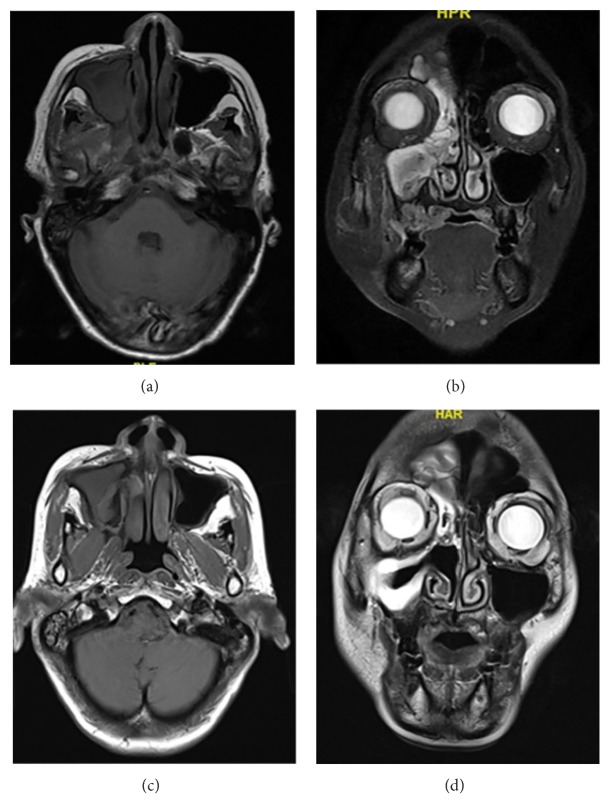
A 64-year-old woman was diagnosed with IMT of the nasopharynx and paranasal sinus. She underwent incomplete resection of tumor, combined with oral prednisone and radiotherapy. After 20-month follow-up, both clinical and imaging manifestations improved. The axial and coronal MRI images before treatment were shown above ((a) and (b)) and that 9 months after radiotherapy were shown below ((c) and (d)) in the figure.
